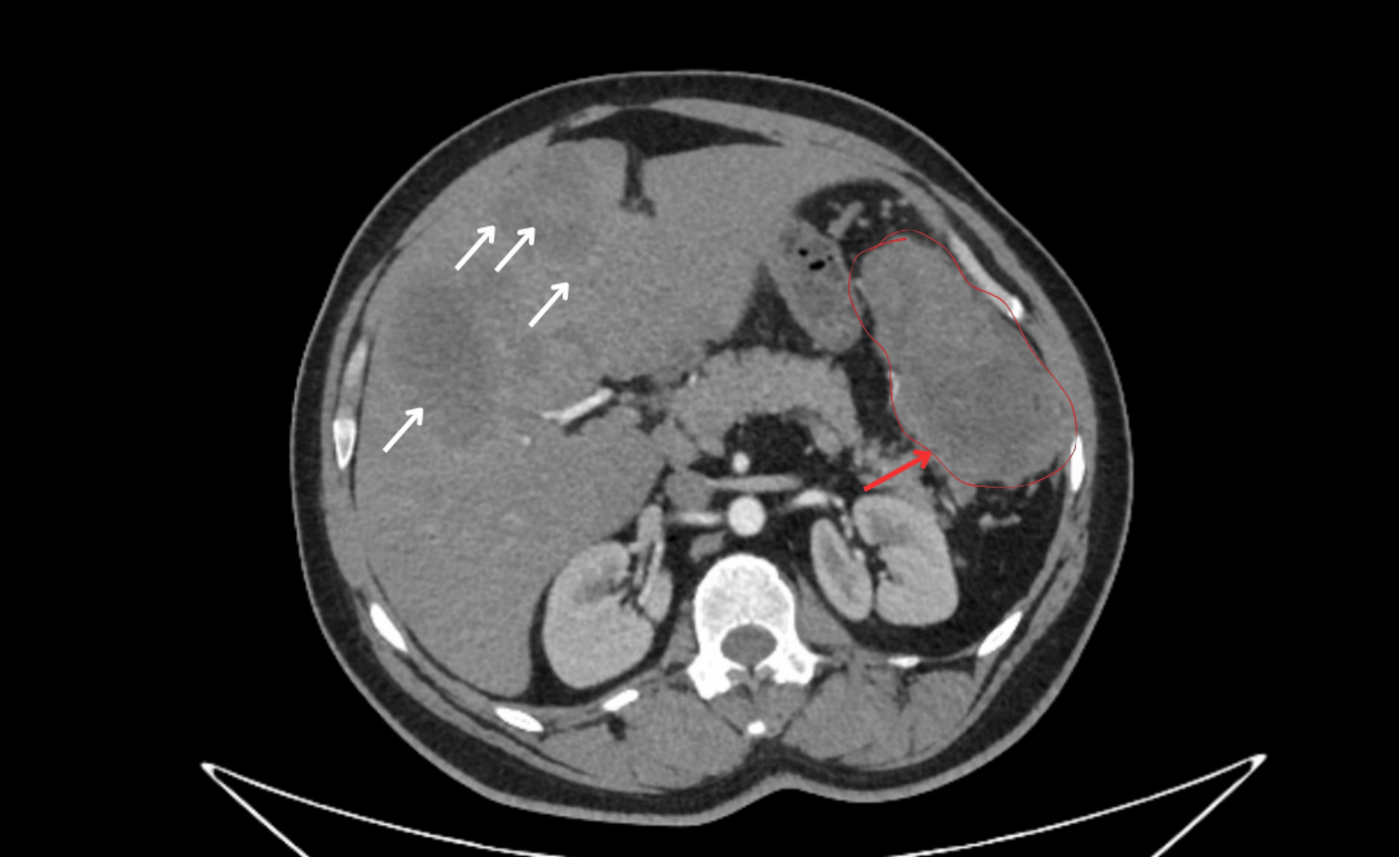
CT Imaging of the DSRCT peritoneal mass with liver metastases. DSRCT: desmoplastic small round cell tumor. Red arrow: Mass attached to the wall of the splenic flexure, measuring 113 × 61 mm. White arrows: Multiple peripheral, continuously enhancing lesions, consistent with liver metastases
